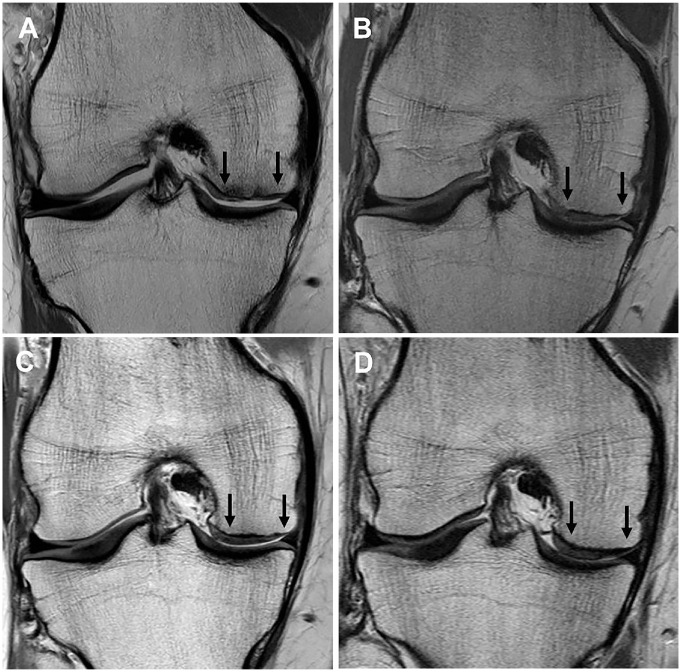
Proton density fast spin-echo magnetic resonance imaging scans of (A) a chondral defect preoperatively on the medial femoral condyle (between black arrows), as well as the subsequent matrix-induced autologous chondrocyte implantation graft at (B) 2 years, (C) 5 years, and (D) 10 years.
